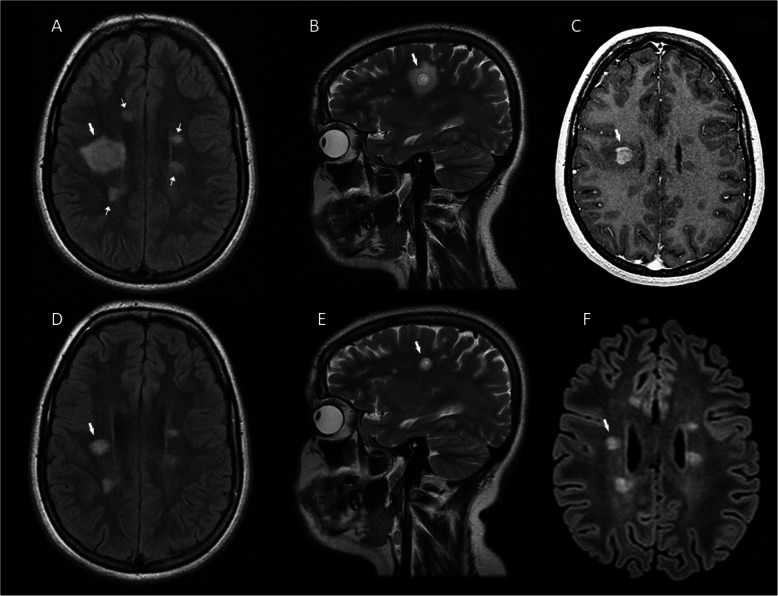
MRI of Case 4. Tumefactive BCS-type lesion in the right centrum semiovale (A and B thick arrow) with incomplete central solid Gd + along with incomplete ring-like Gd + of the outer two layers (C thick arrow) and multiple typical non-enhancing MS lesions supratentorially (A thins arrows). BCS-type lesion with effacement of the “onion bulb” pattern (D and E thick arrow) 2 months after BCS onset and after corticosteroid treatment. BCS-type lesion (F thick arrow) at the 7th month of natalizumab treatment and 13th from the onset. a, d: FLAIR images. b, e: T2-weighted images. c: T1-weighted contrast-enhanced images. f: 3D FLAIR image. BCS: Baló’s concentric sclerosis, Gd+: gadolinium enhancement
